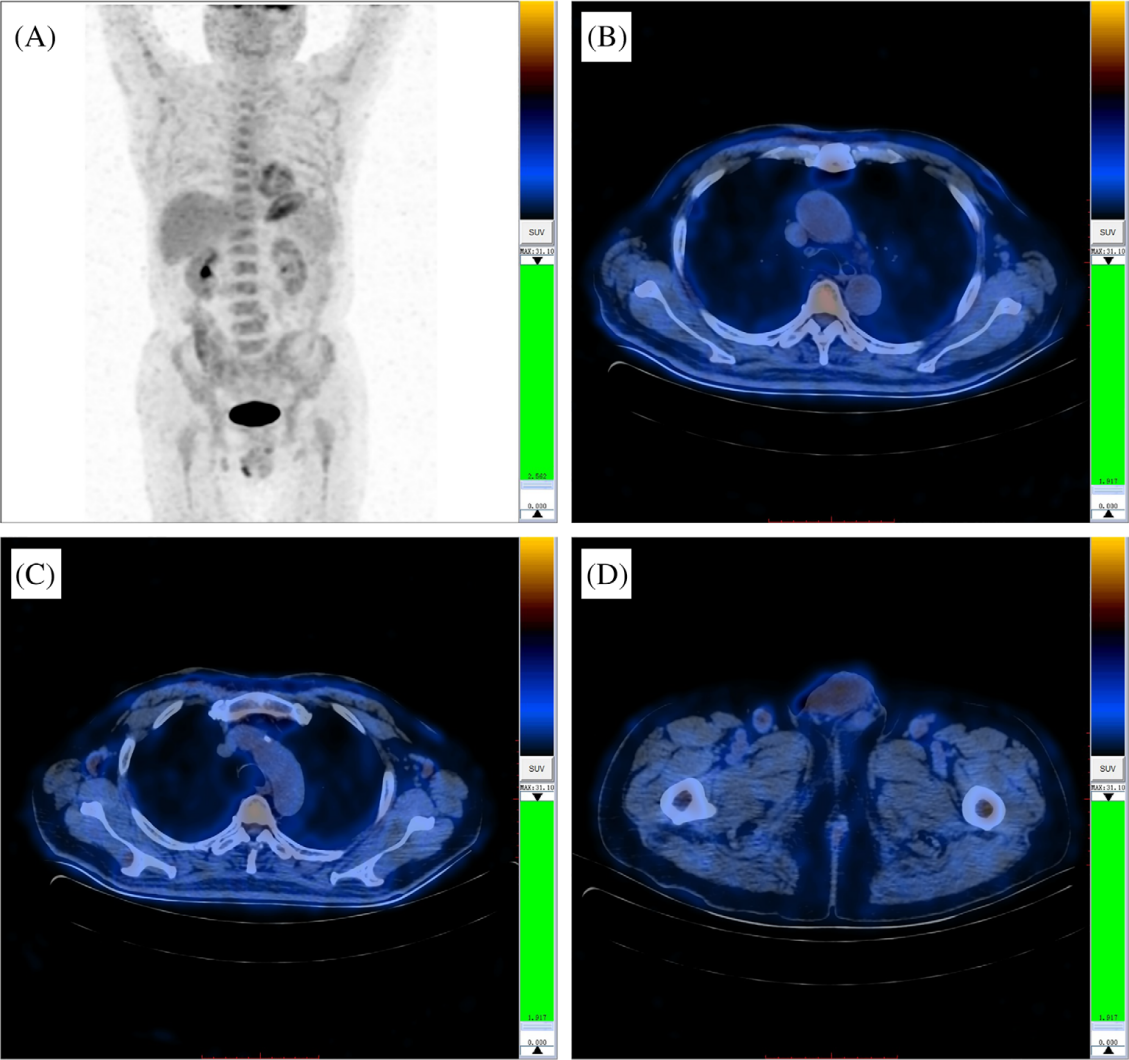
Images of FDG PET‐CT. A, PET maximum intensity projection (MIP) image suggested multiple mild FDG‐avid cutaneous lesions and involvement of lymph nodes and trunk bone. B, PET‐CT fusion image showed thickened and mild FDG‐avid cutaneous lesions on the chest. C and D, PET‐CT fusion images revealed involvement of bilateral axillary and inguinal lymph nodes [Colour figure can be viewed at wileyonlinelibrary.com]
